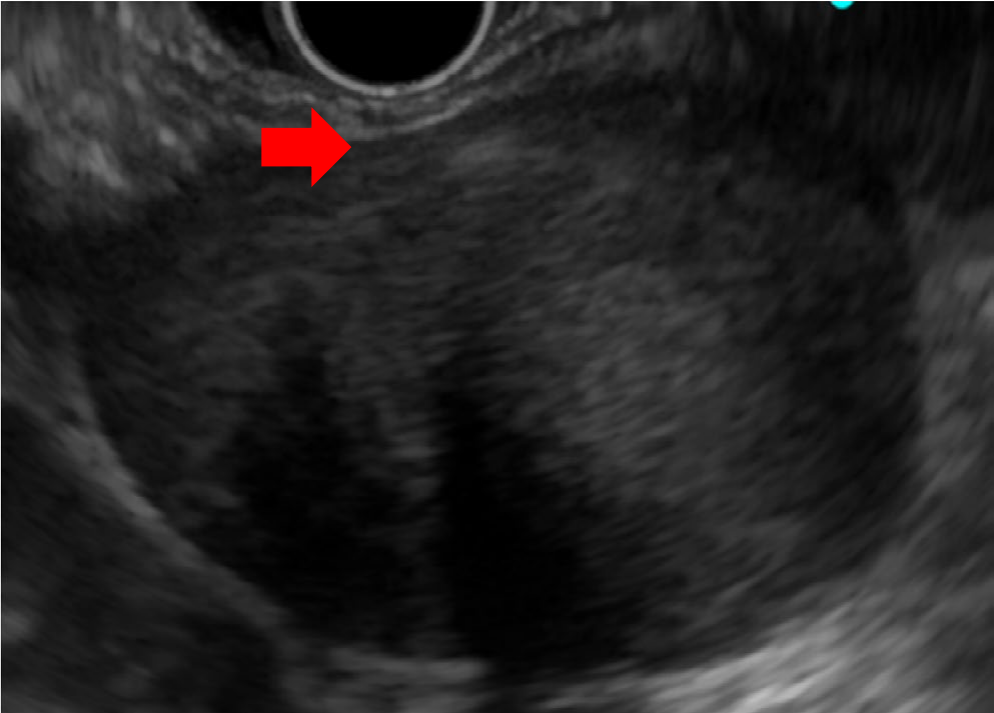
Endoscopic ultrasound image. A 47.3 × 31.3‐mm hypoechoic tumor at the posterior wall of the middle part of the gastric corpus. It is suspected to be derived from the muscular layer of the fourth layer of the gastric wall (red arrow)
